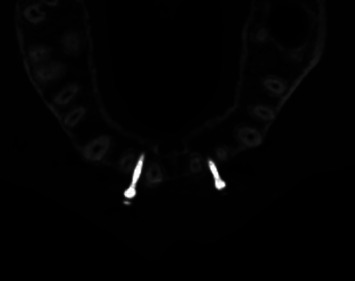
Computed tomography scan of the anterior maxillary area immediately after implantation.
